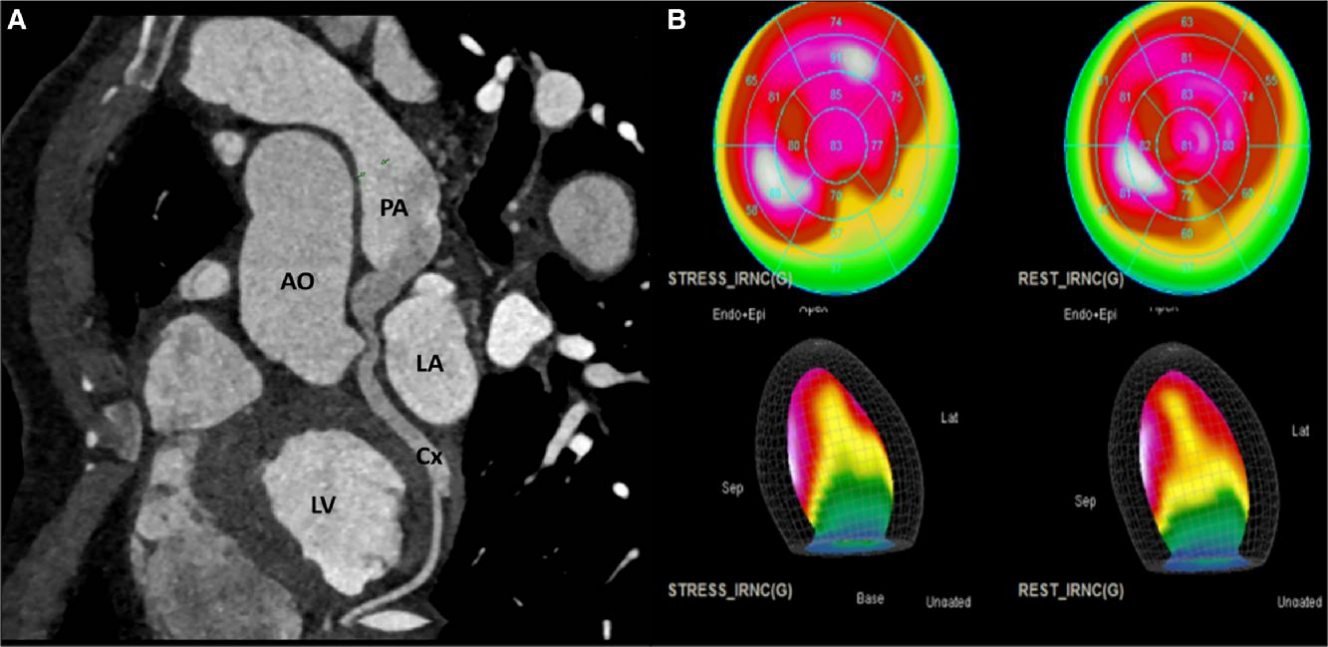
(*A*) Gated cardiac computed tomography: curved multiplanar reconstruction shows the abnormal origin of the circumflex artery from the pulmonary artery with aneurysmal deformation on the draining area. (*B*) Stress single photon emission computed tomography shows ischaemia in three latero-basal segments.
